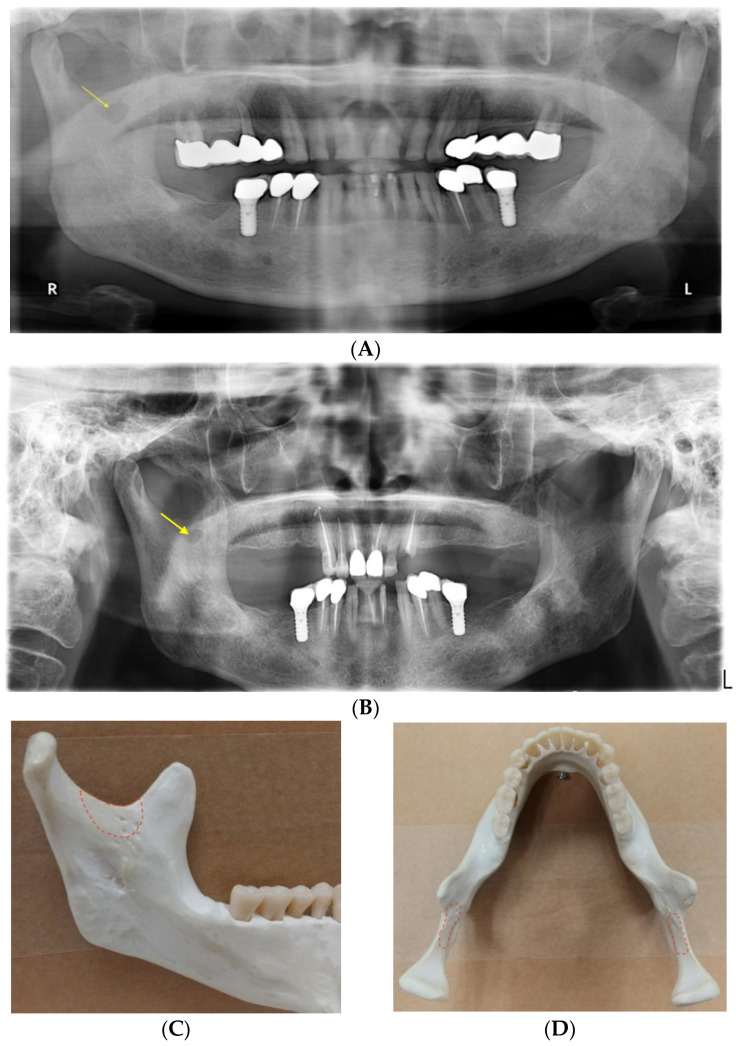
Dental panoramic radiographs showing a medial sigmoid depression (MSD) on the right side of the mandible, taken in (A) 2012 and (B) 2021, respectively. Its appearance did not change much between two timepoints. A mandible model showing the location where an MSD may be present from the (C) lingual and (D) axial views.
